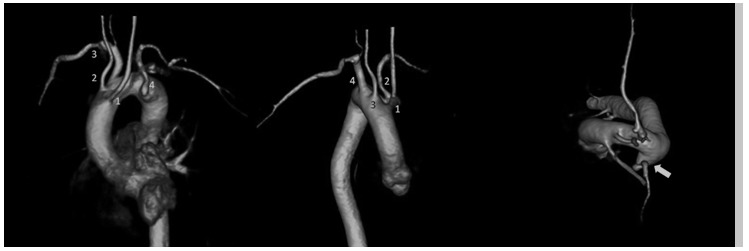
MRI Angiography of the thoracic aorta Volume rendering images. Anterior (*on the left)*, right (*at the center*) and superior (*right*) views:1: left common carotid artery; 2: right common carotid artery; 3: right subclavian artery; 4: left subclavian artery originating from the Kommerell’s diverticulum (arrow).
